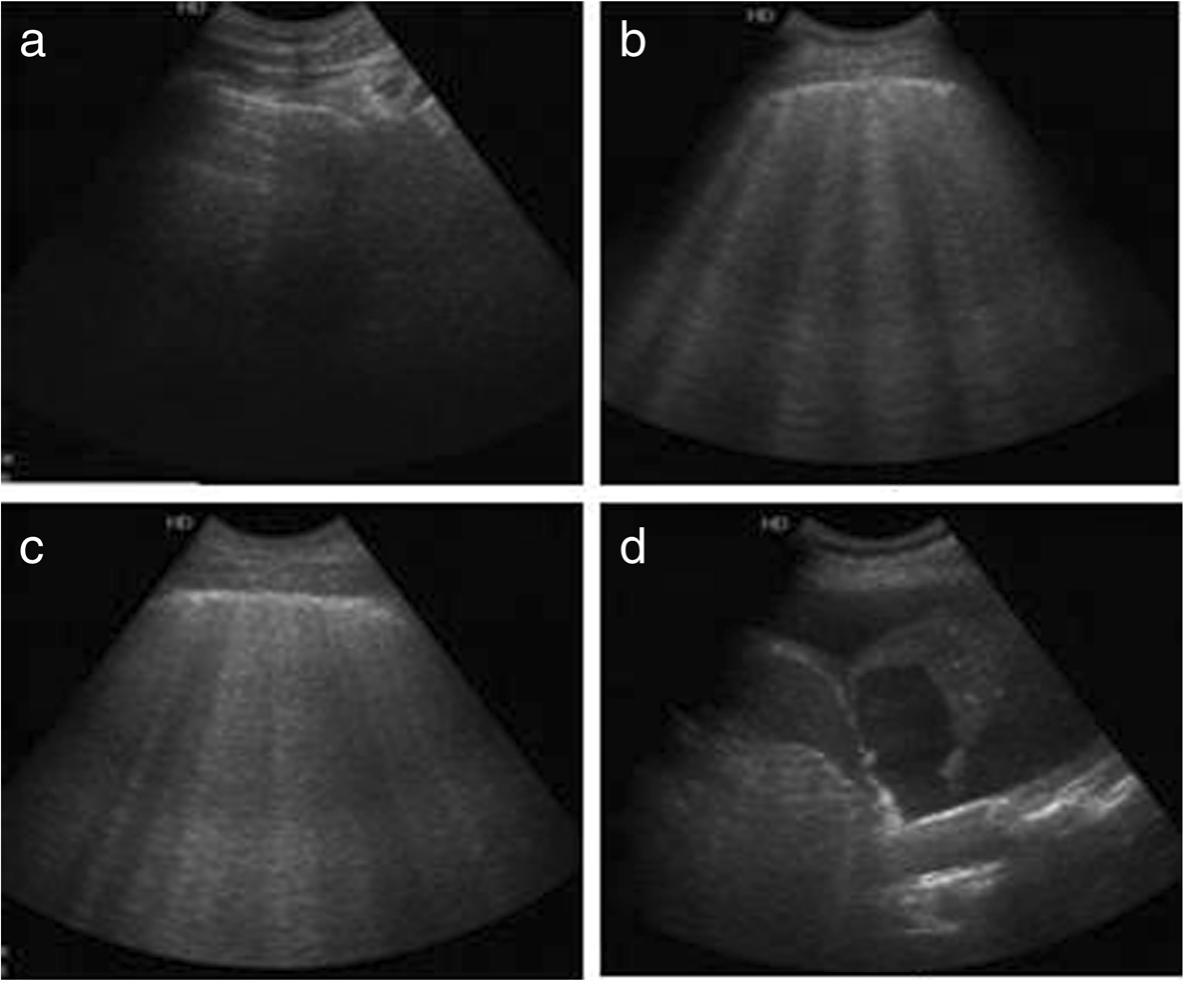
Four ultrasound patterns according to lung aeration. a normal aeration (N): presence of lung sliding with A lines or fewer than two isolated B lines; b moderate loss of lung aeration: multiple, well-defined B lines (B1 lines); c severe loss of lung aeration: multiple coalescent B lines (B2 lines); and d lung consolidation (C), the presence of a tissue pattern. For a given region of interest, points were allocated according to the worst ultrasound pattern observed: N = 0, B1 lines = 1, B2 lines = 2, C = 3
